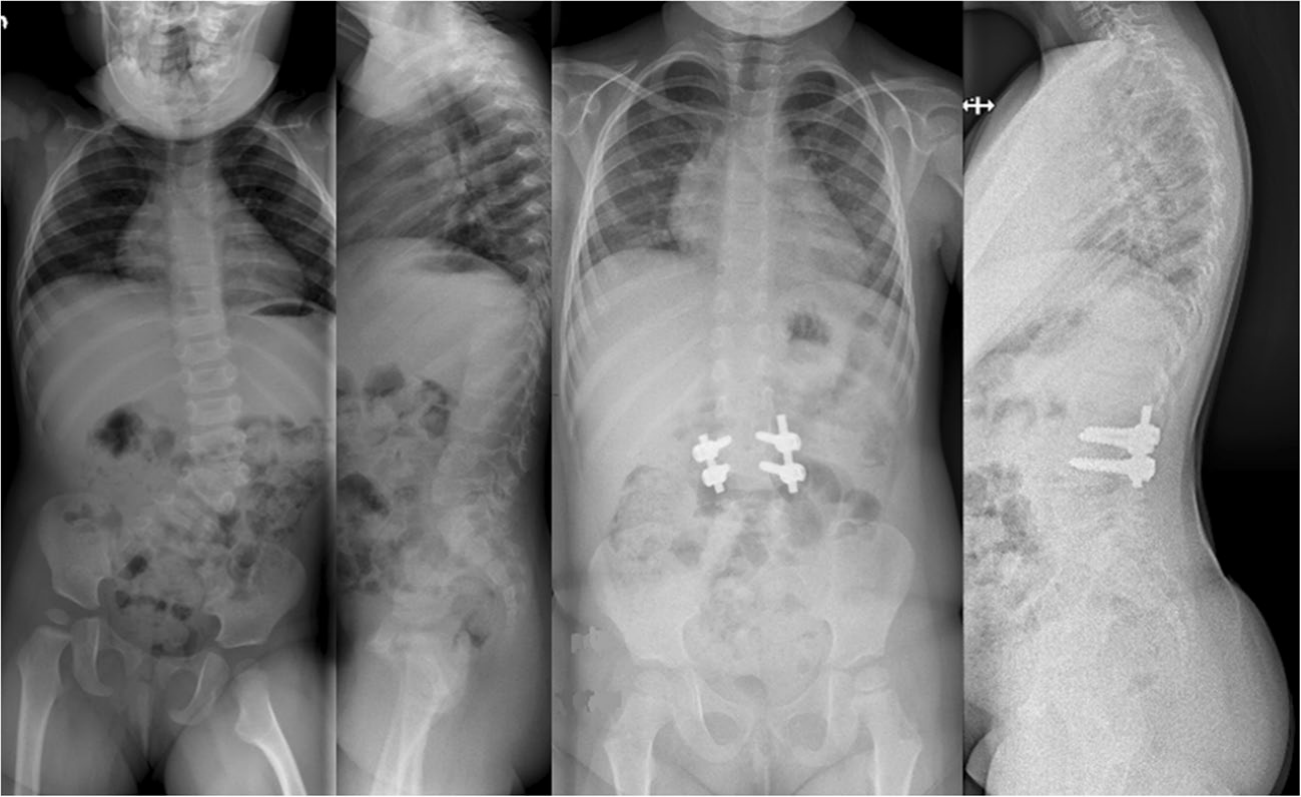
An 18-month-old girl with congenital scoliosis due to L3 posterolateral hemivertebra. L3 hemivertebrectomy with short-instrumented fusion from L2 to L4 with intraoperative blood loss of 170 ml. Standing posteroanterior and lateral preoperative and 2-year follow-up radiographs
